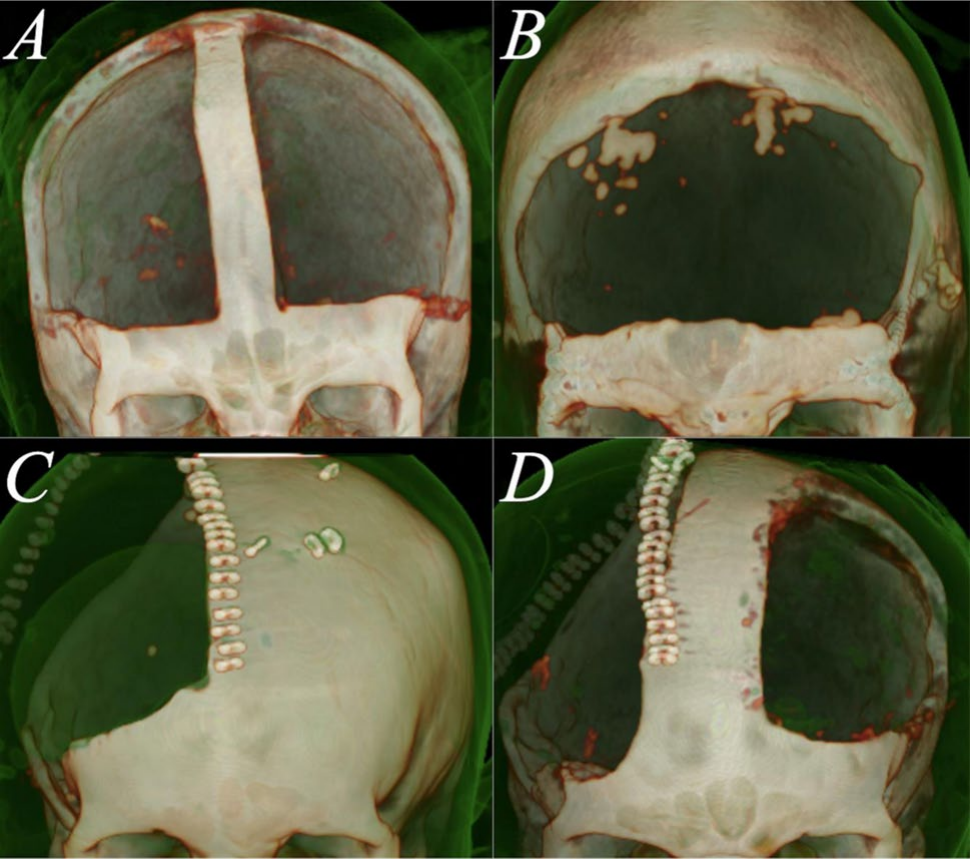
Three-dimensional computed tomography reconstructions of the four types of decompressive craniectomy performed in our centre. A depicts a bifrontal two-flap craniectomy, B a bifrontal one-flap craniectomy, C a unilateral frontotemporoparietal hemicraniectomy and D a bilateral hemicraniectomy. In B, dural osteogenesis is seen at the craniectomy defect site (scan taken 2 months after DC)
